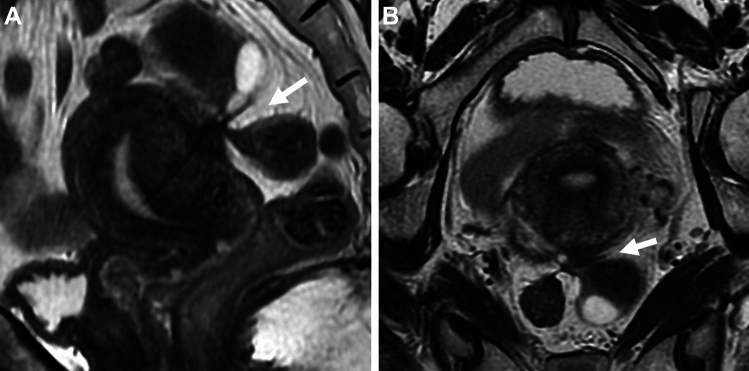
Deep endometriosis. A 48-year-old with a history of pelvic pain and suspicion of deep posterior endometriosis. A Sagittal and B oblique coronal T2-weighted images show the elevation of the posterior vaginal fornix, low signal intensity fibrotic plaque with stellate margins (arrow) on the serosal surface of the retroflexed uterus. Tethered appearance of the rectum to the uterus with low signal intensity faint fibrous strands is seen
